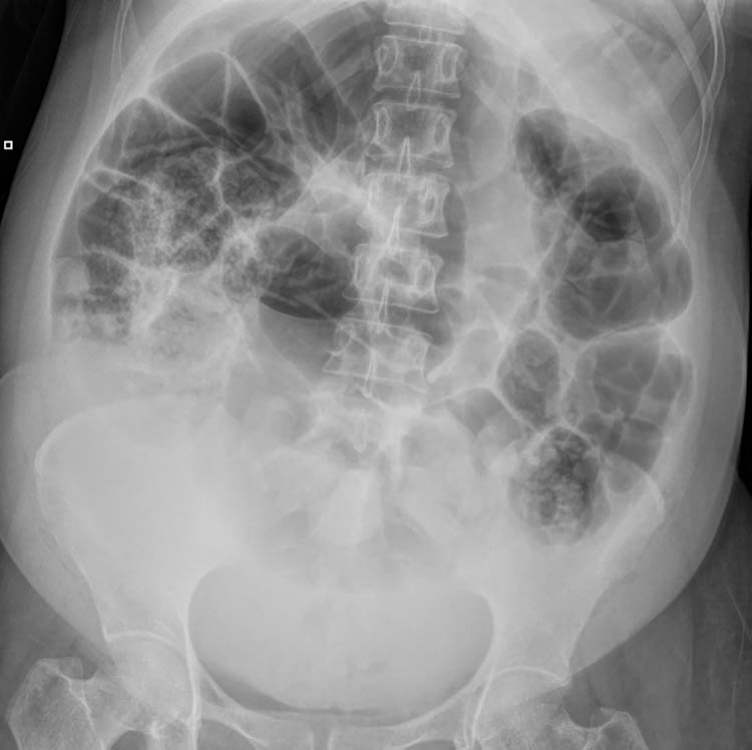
X-ray Abdomen, 24 h after commencement of symptoms. Widespread dilatation of large and small intestines stopping at the level of the pelvic brim. Moderate faecal loading in the caecum and descending colon.
